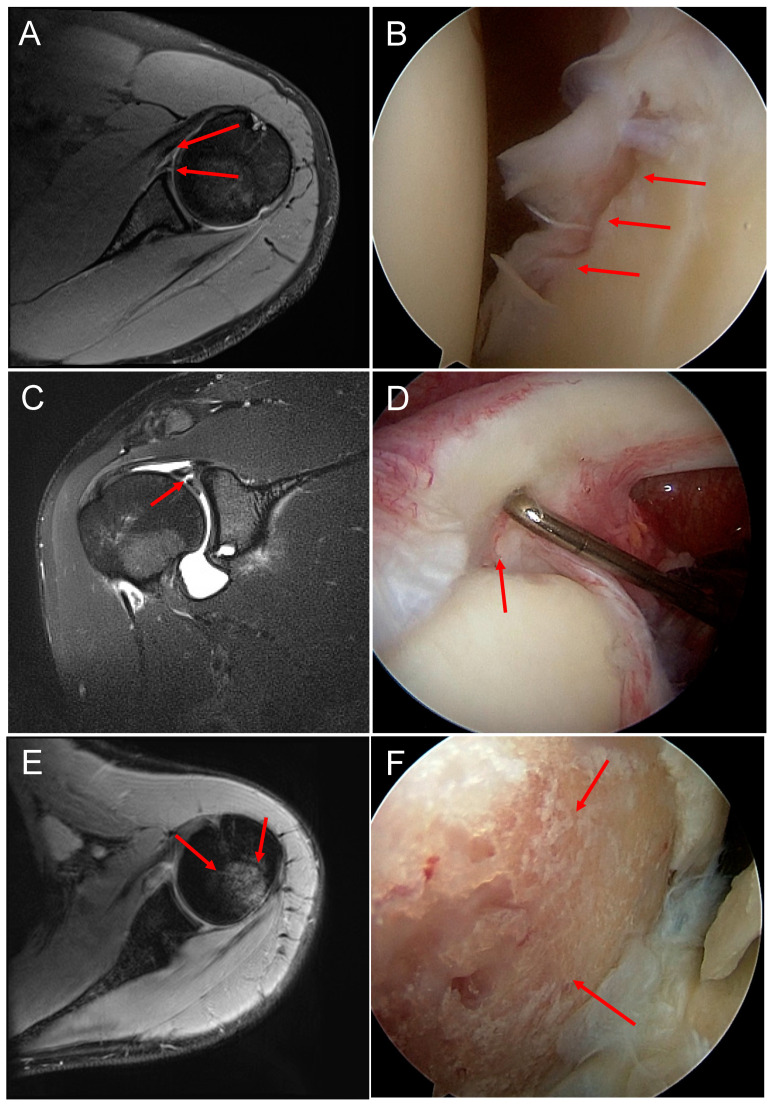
(A) MR image of a Bankart lesion (red arrows). (B) Corresponding arthroscopic image of a Bankart lesion. (C) MR image of a SLAP lesion. (D) Corresponding intraoperative image of a SLAP lesion. (E) MR image of a Hill–Sachs lesion. (F) Arthroscopic image of a Hill–Sachs lesion.
